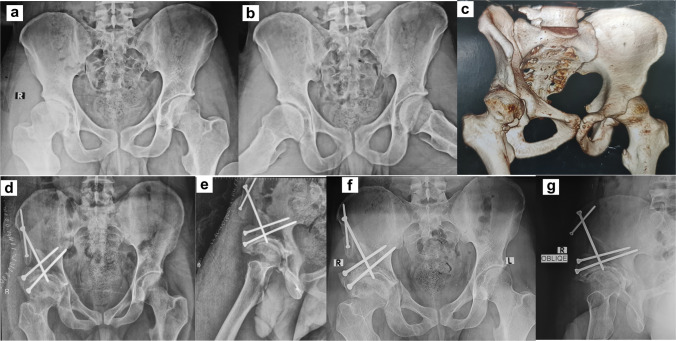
A 28-year-old male with symptomatic adult acetabular dysplasia, more pronounced on the right hip, treated with PAO. a–c Preoperative AP and lateral hip radiograph with 3D CT demonstrating reduced lateral center–edge angle (LCEA) and increased acetabular index (AI) consistent with acetabular dysplasia. d, e Immediate postoperative AP pelvic and lateral hip radiographs showing adequate acetabular reorientation with correction of both LCEA and AI, stabilized using four 4.5-mm cortical screws. f, g Final follow-up radiographs at 7 years demonstrating maintained acetabular correction, complete osteotomy union, stable fixation, and absence of secondary complications
